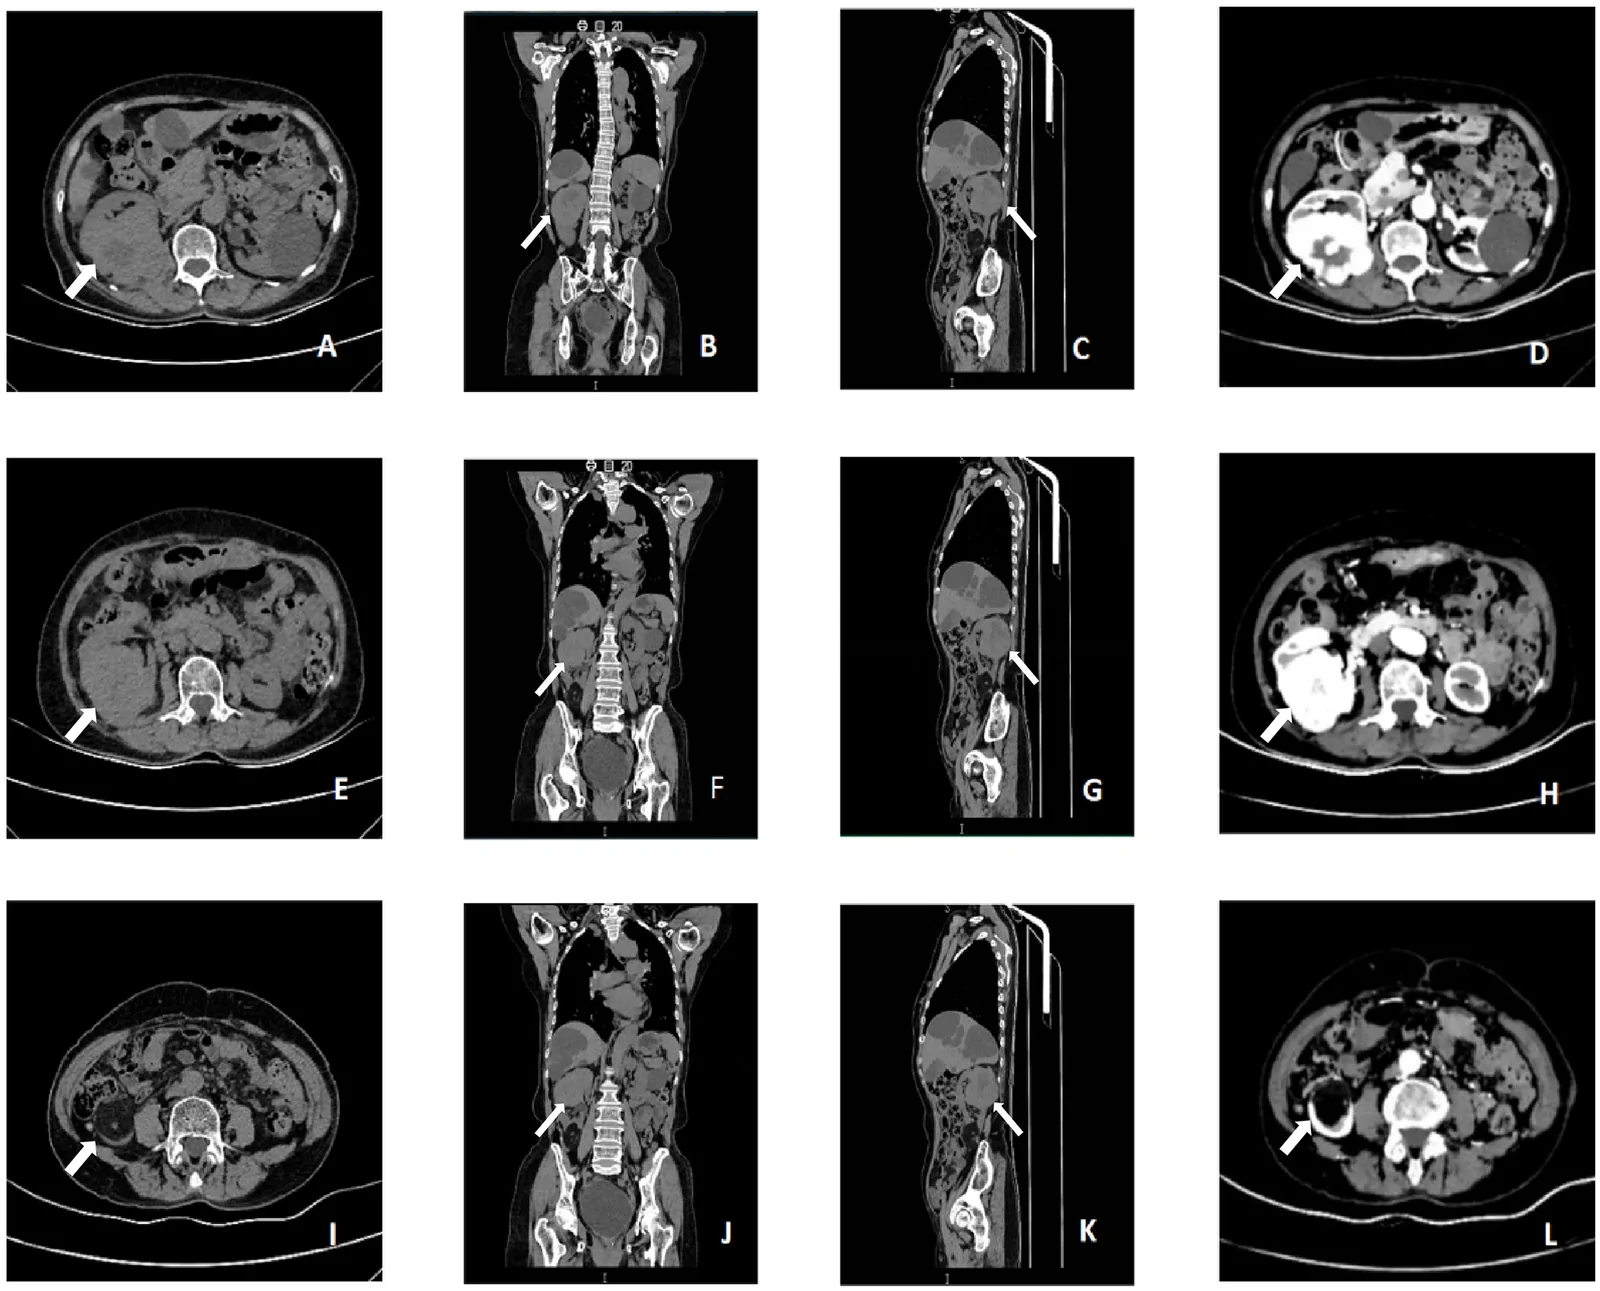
CT urography (CTU) of the right kidney demonstrating three discrete lesions with different enhancement patterns. (A–D) Upper-pole mass: axial, coronal, and sagittal noncontrast images and the corticomedullary (arterial) phase show an irregular mixed-attenuation lesion with marked heterogeneous enhancement (white arrows). (E–H) Hilar mass: corresponding multiplanar noncontrast and corticomedullary-phase images demonstrate a mixed-attenuation lesion with avid enhancement (white arrows). (I–L) Lower-pole lesion: multiplanar images show a round low-attenuation mass with focal enhancement in the corticomedullary phase and areas of cystic necrosis also visible (white arrows).
